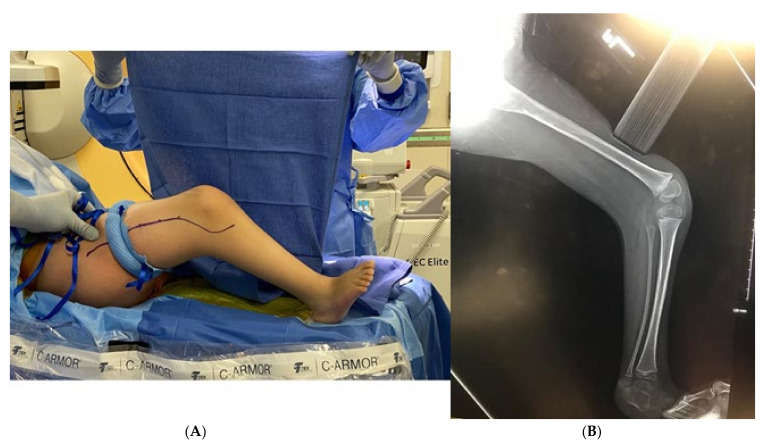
(A) Example of incision placement on the lateral thigh. (B) Maximum extension radiograph demonstrating 70° knee flexion contracture.
